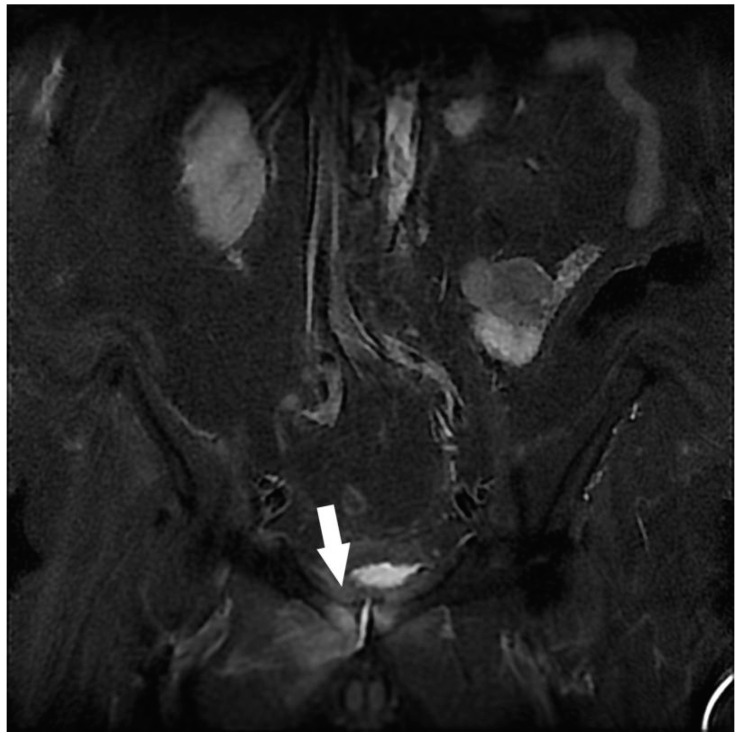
Assessment of right sciatica in an 80-year-old man two months after prostatectomy. Coronal STIR MRI. Hypersignal in mirror with effusion of the pubic symphysis. Inflammatory hypersignal in the proximal part of the adductor muscles, predominant on the right (white arrow). Septic pubic arthritis (confirmed by subsequent biopsy).
